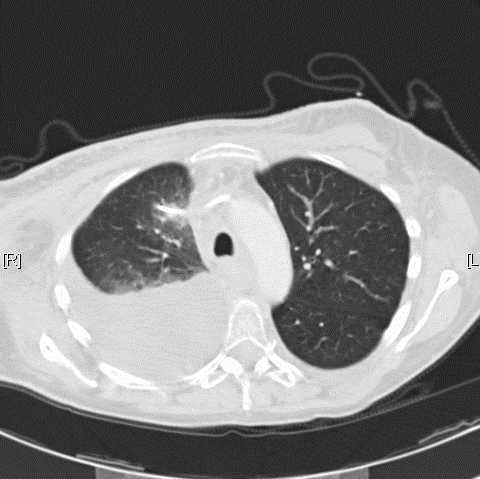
CT scan showing the catheter tip in the right upper lobe of the lung and a large dorsobasal pleural effusion.
